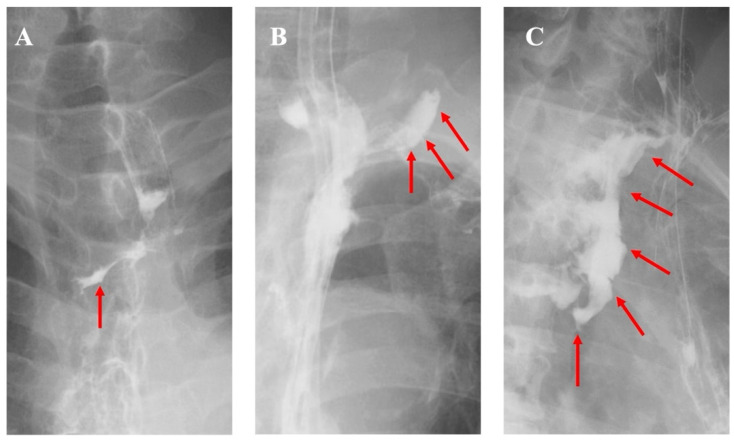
Radiographic grading of anastomotic leakage. (A) Grade I: linear contrast leakage without cavity formation. (B) Grade II: localized cavity near the anastomosis. (C) Grade III: leakage extending into the mediastinum or thoracic cavity. Red arrows indicate extraluminal leakage of contrast medium.
